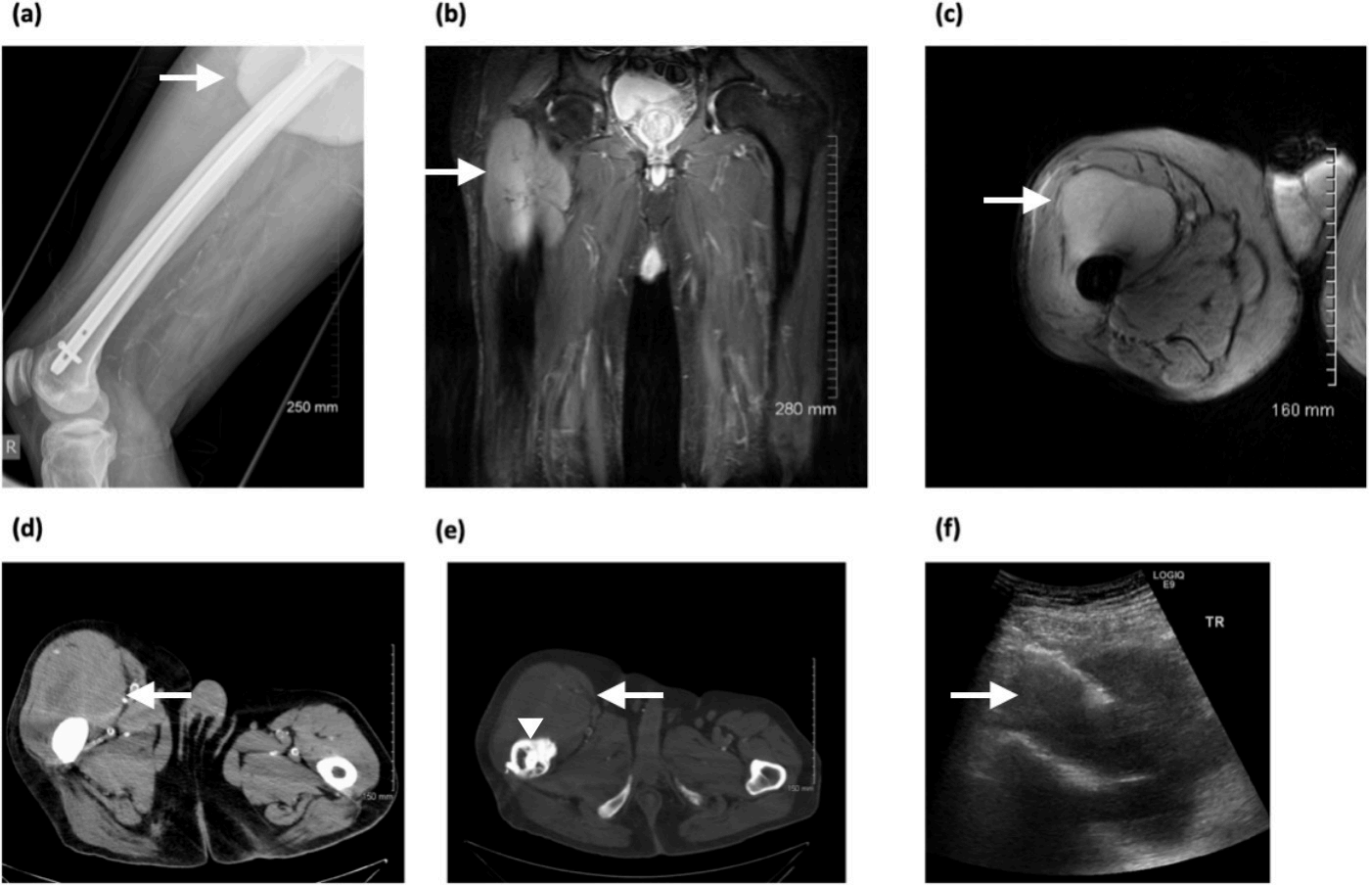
(a) Lateral radiograph of right femur showing an area of increased density in the upper right thigh, (b, c) coronal and axial *T*_2_ weighted MR images showing large 18 × 6 cm hyperintense mass in upper lateral thigh which is well defined and non-infiltrative, (d, e) axial CT images showing the mass (arrows) and periosteal reaction (arrow head), (f) sonogram during biopsy of the mass.
